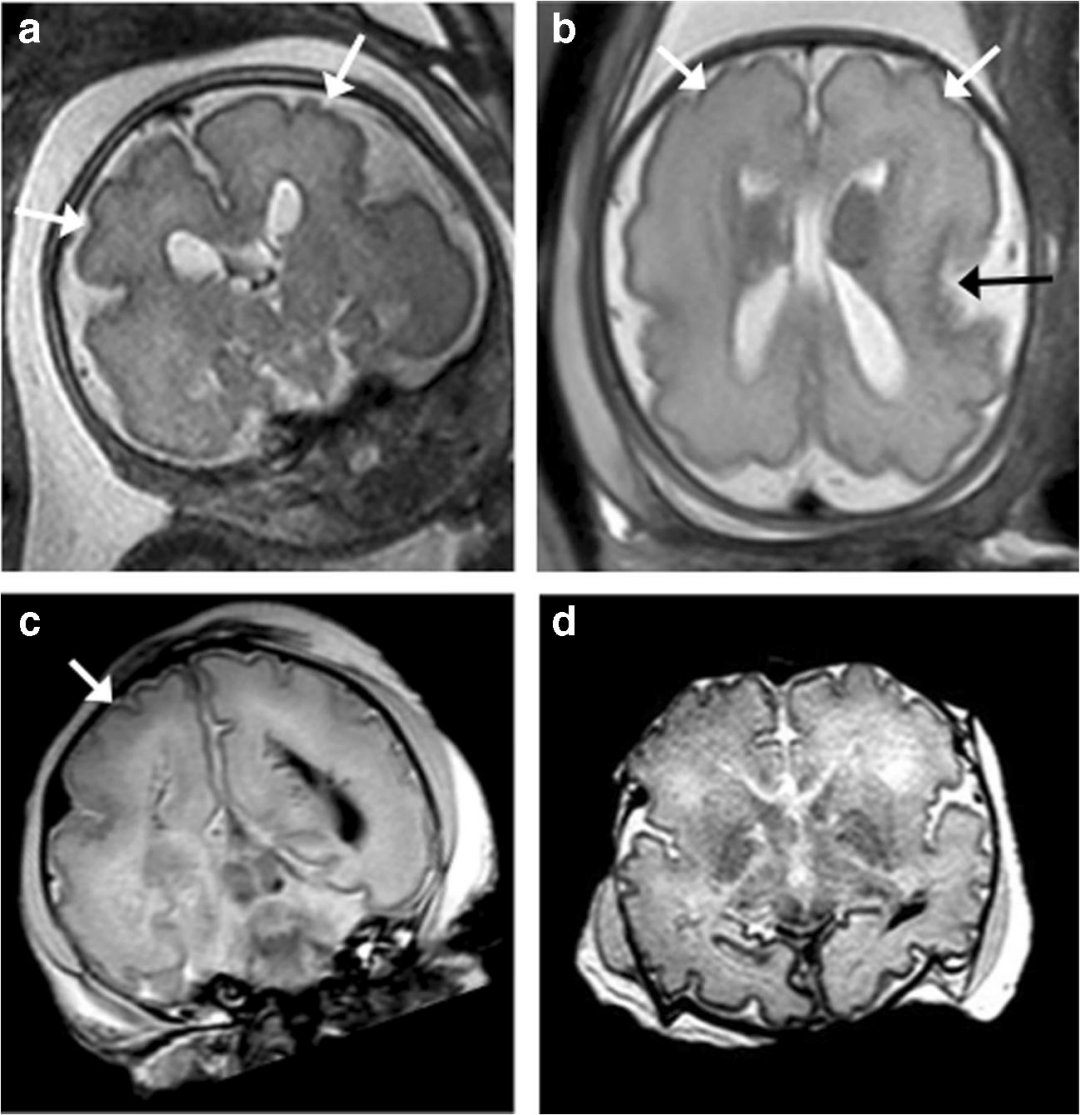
Megalencephaly polymicrogyria polydactyly and hydrocephalus (MPPH) syndrome due to PIK3R2 mutation in a 29-week gestation fetus. a Coronal and b axial T2-weighted iuMR images demonstrate bilaterally enlarged cerebral hemispheres and perisylvian polymicrogyria (white arrows). There was also a misshapen Sylvian fissure (black arrow). c Coronal and d axial T2-weighted PMMR images also demonstrate polymicrogyria (white arrows), particularly of the right cerebral hemisphere although this was harder to appreciate than on iuMR
